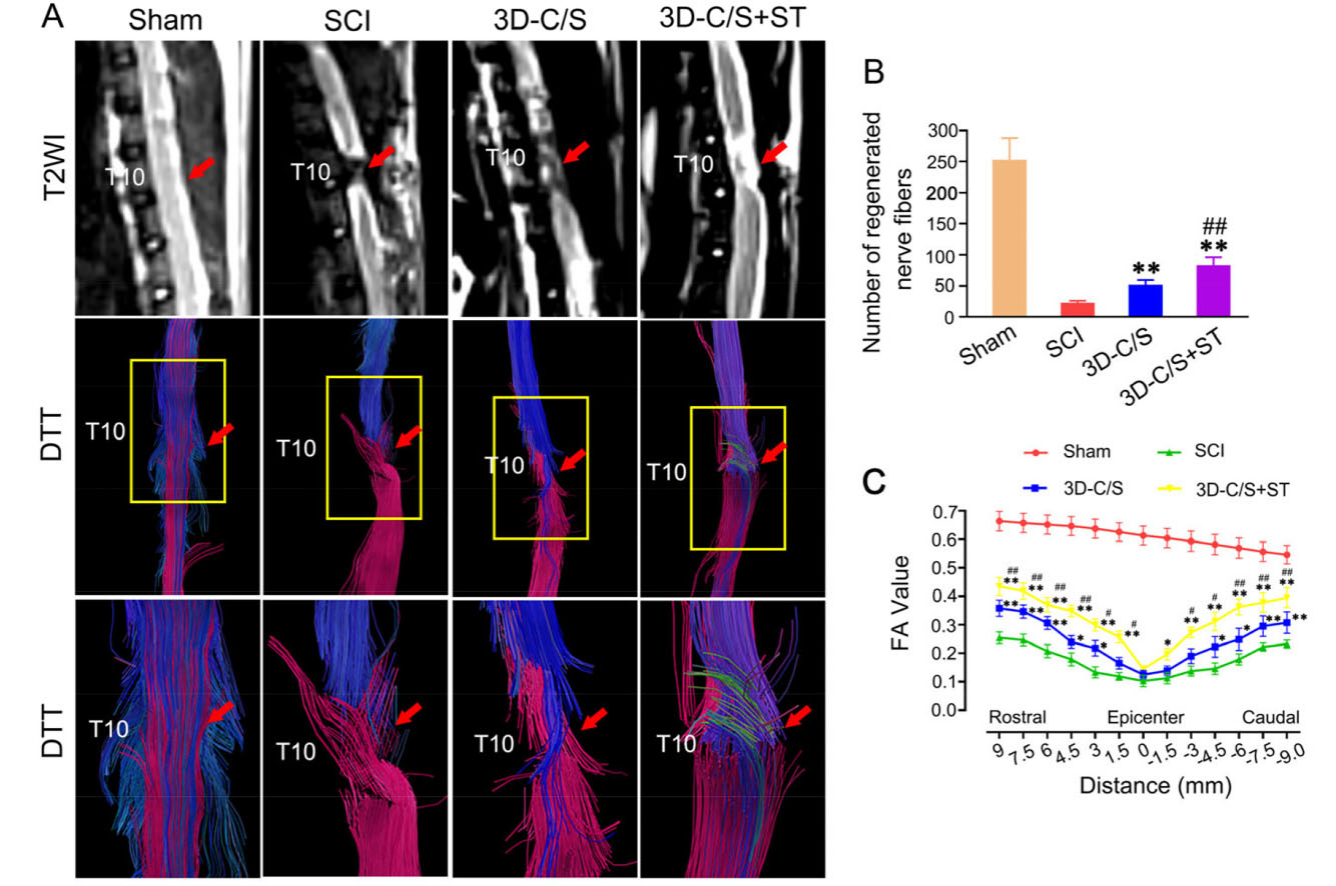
MRI and DTI at 8 weeks after surgery. (A) Typical MRI T2 images and DTT images. The yellow box regions in the Middle image were enlarged in the below image. (B) Quantification of regenerated nerve fibers at the injury area. (C) Distribution map of FA value corresponding to the injury area. P *<* *0.05, *P *<* *0.01 vs SCI. ^#^*P *<* *0.05, ^##^*P *<* *0.01 vs 3D-C/S.
